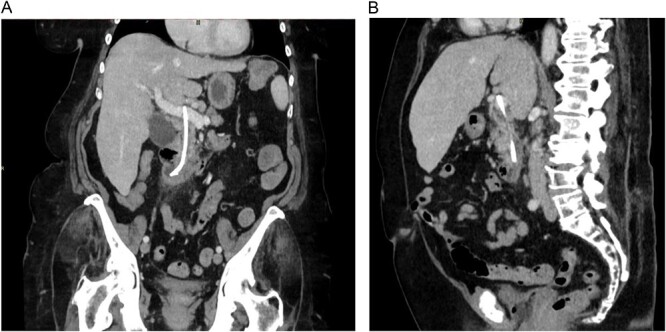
CT images showing the migrated common bile duct stent in the portal vein. (A) Coronal view. (B) Sagittal view.
